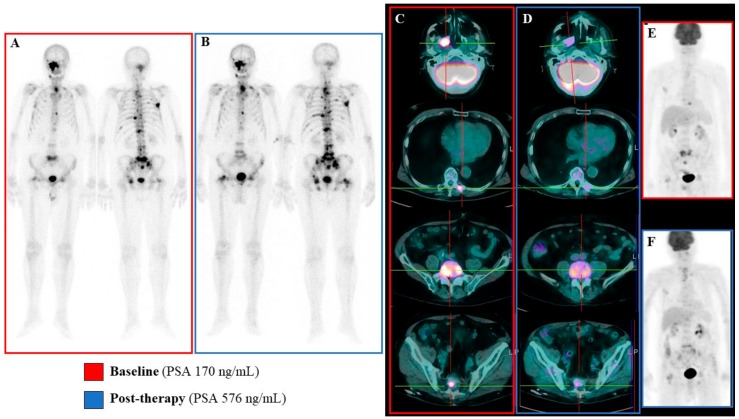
Emblematic example of mismatch between metabolic and biochemical/bone scan response. In this emblematic case a mismatch between metabolic data and biochemical/bone scan results was observed. At baseline the bone scan revealed the presence of several osteoblastic bone lesions (Panel A) that were mainly FDG-avid (Panels C and E). The post-therapy evaluation, performed six weeks after Ra223 protocol completion, showed the persistence of osteoblastic bone lesions that were occasionally more extended and diphosphonate-avid (Panel B). This finding was paralleled by increased serum PSA levels (576 vs 170 ng/mL at baseline). However, the post-therapy FDG PET/CT showed a significant reduction in bone lesions FDG uptake, leading to a Partial Metabolic Response according to PERCIST criteria (Panels D and F).
